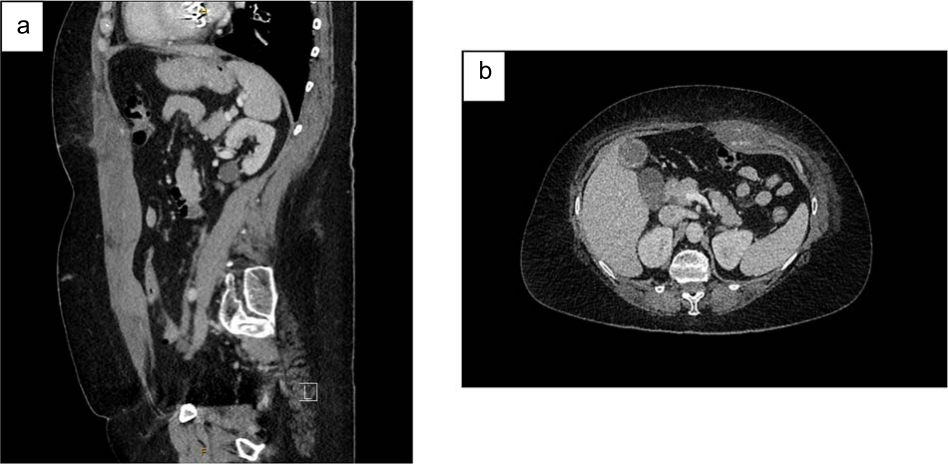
Contrast CT abdomen and pelvis (portal venous phase) demonstrating a left-sided RSH with active extravasation: (a) Sagittal view; (b) Axial view.
